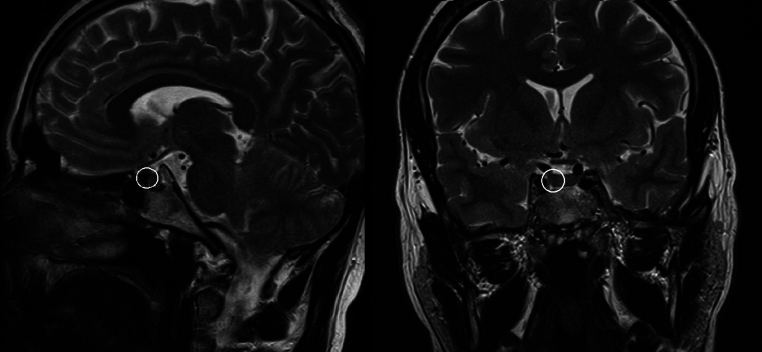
Pituitary MRI T2-weighted coronal and sagittal views where a small area (highlighted) at the right bottom of the gland, consistent with a microadenoma, is observed. The lesion was more evident on T2-weighted sagittal views.
